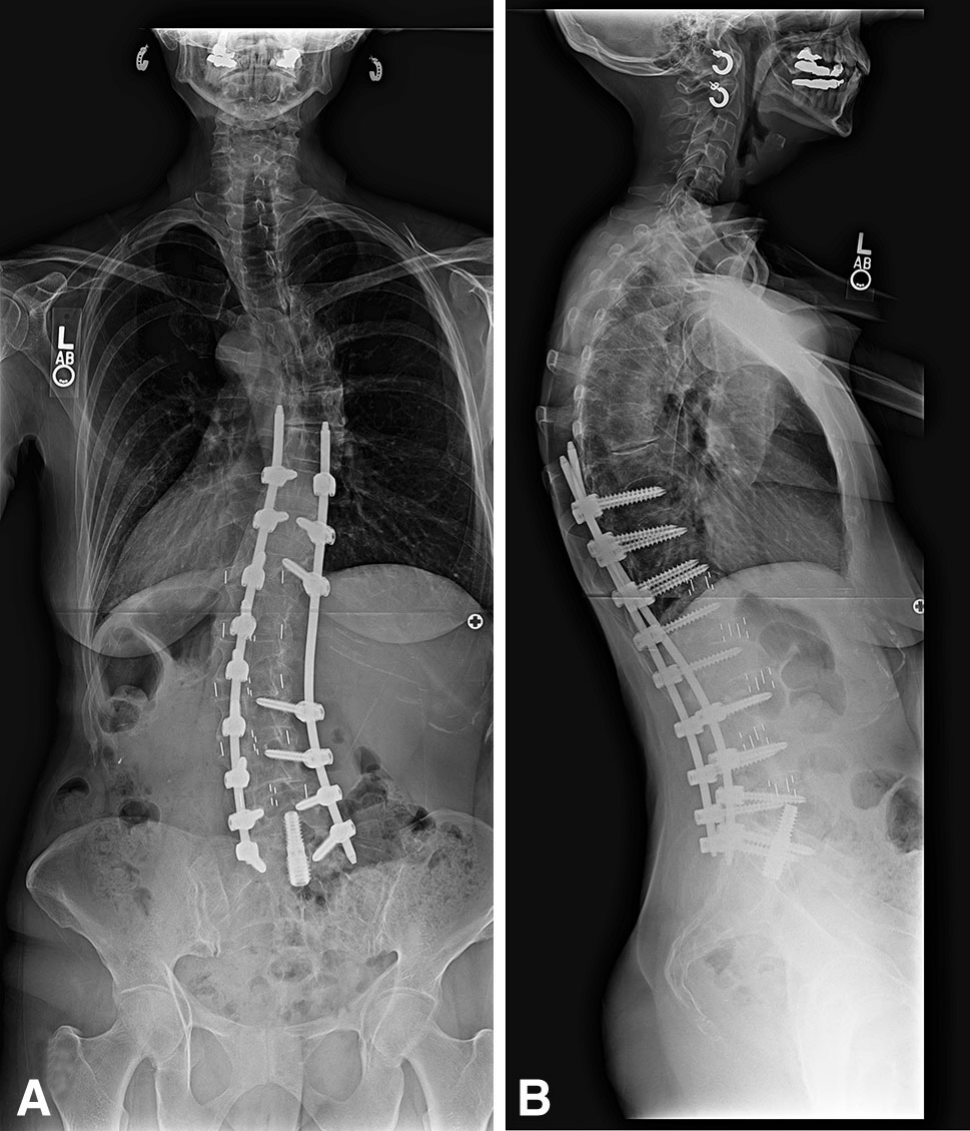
These 36-inch (A) AP and (B) lateral standing radiographs show the spine of the patient in Figure 1 at 3 years after lateral transpsoas discectomy and interbody fusion, percutaneous pedicle screw and rod placement, and L5-S1 transsacral discectomy and interbody fusion. A solid fusion was achieved at L5-S1 without iliac fixation.
